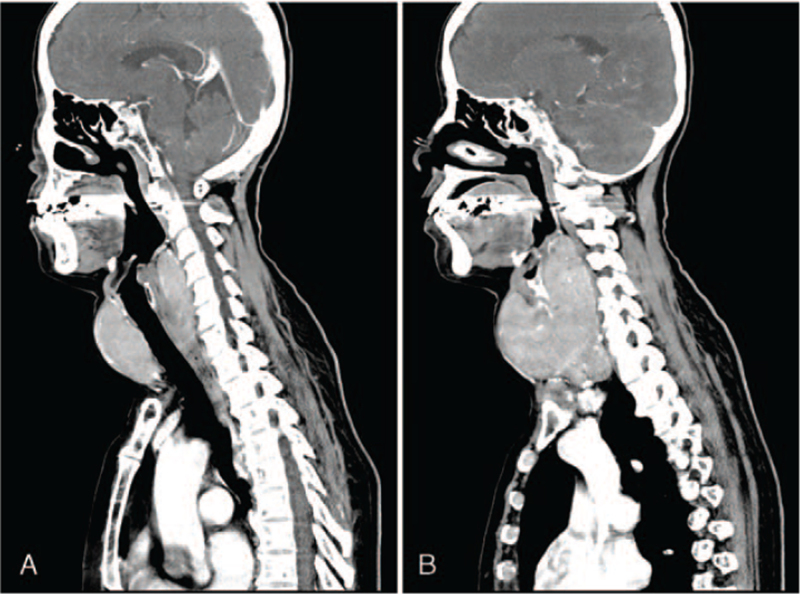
The thyroid goiter that extended from the C3 level to the T1 level and occupied the retropharyngeal space, resulting in anterior displacement of the larynx is shown in the sagittal view of neck computed tomography. An image of the midline plane is shown in (A), and the image of the plane that was shifted slightly to the right from the midline is shown in (B).
